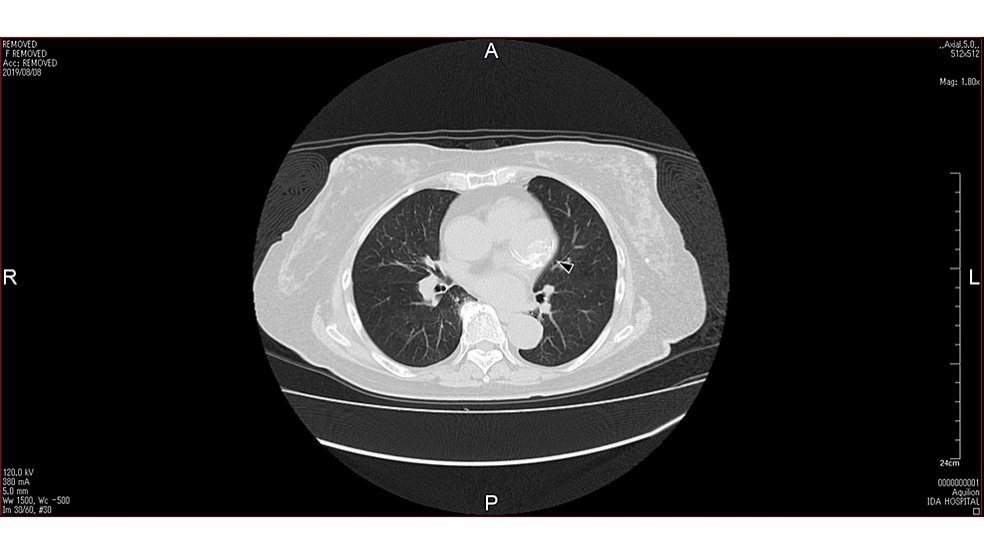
Computed tomography Computed tomography shows a spherical mass (black arrow) with 40 mm of calcification in the cardiac sac outside the heart and next to the PA. PA: pulmonary artery
